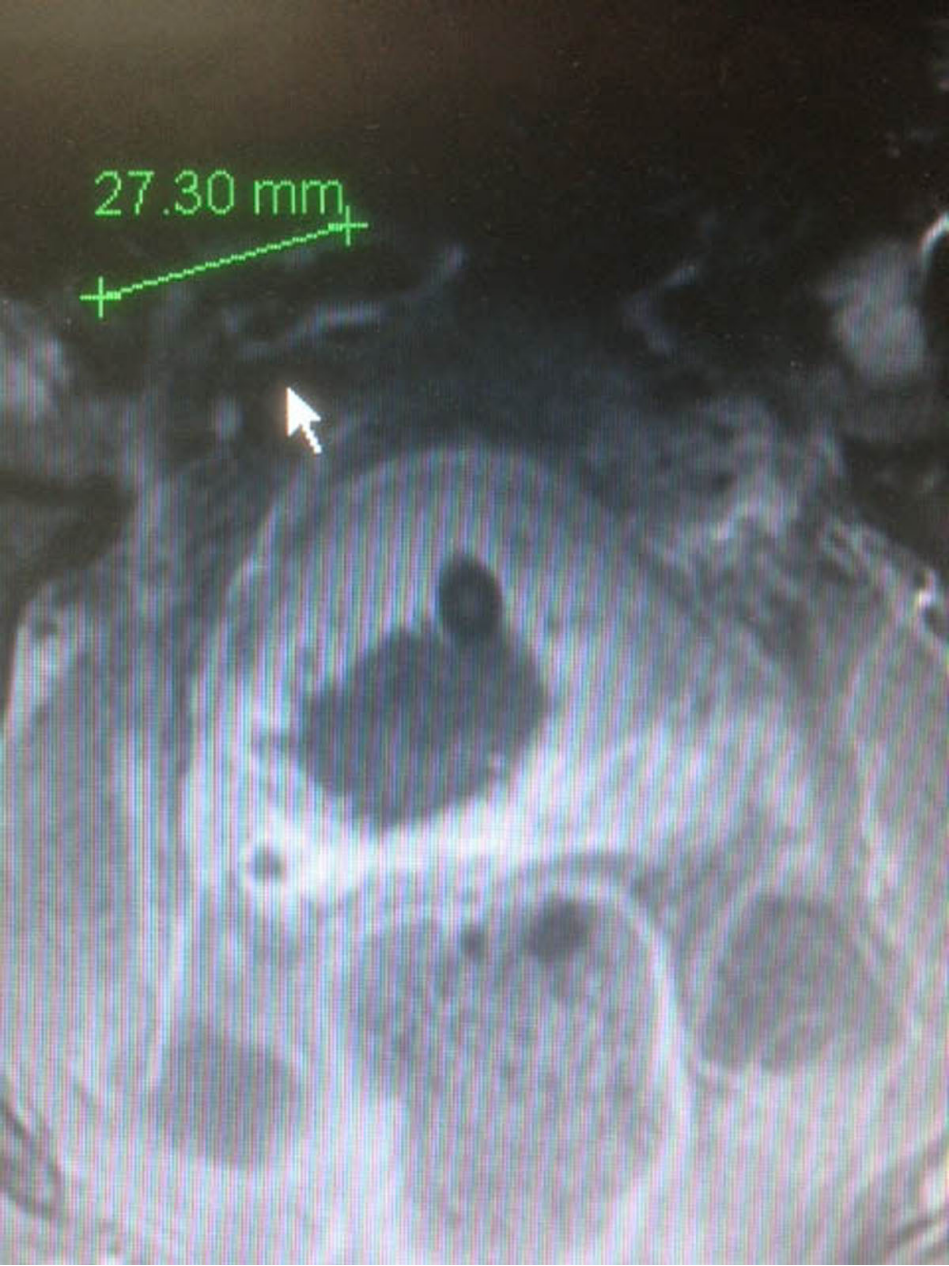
Control MRI after focal HIFU
